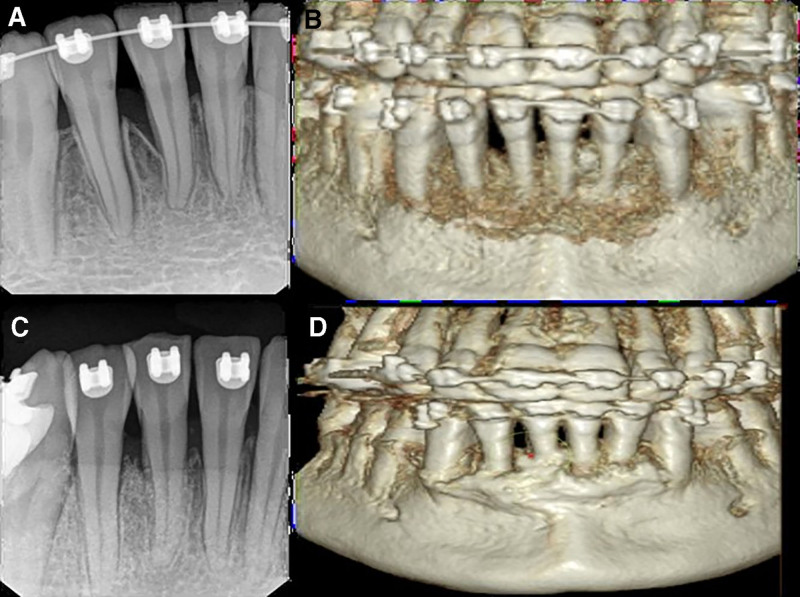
(A) Pre-periapical radiograph showing the bone reduction in the mandibular anterior region (20%–30%); (B) Pre-3D scan CBCT; (C) Post-periapical radiograph showing bone fill; (D) Postoperative 3D scan CBCT. CBCT = cone beam computed tomography.
